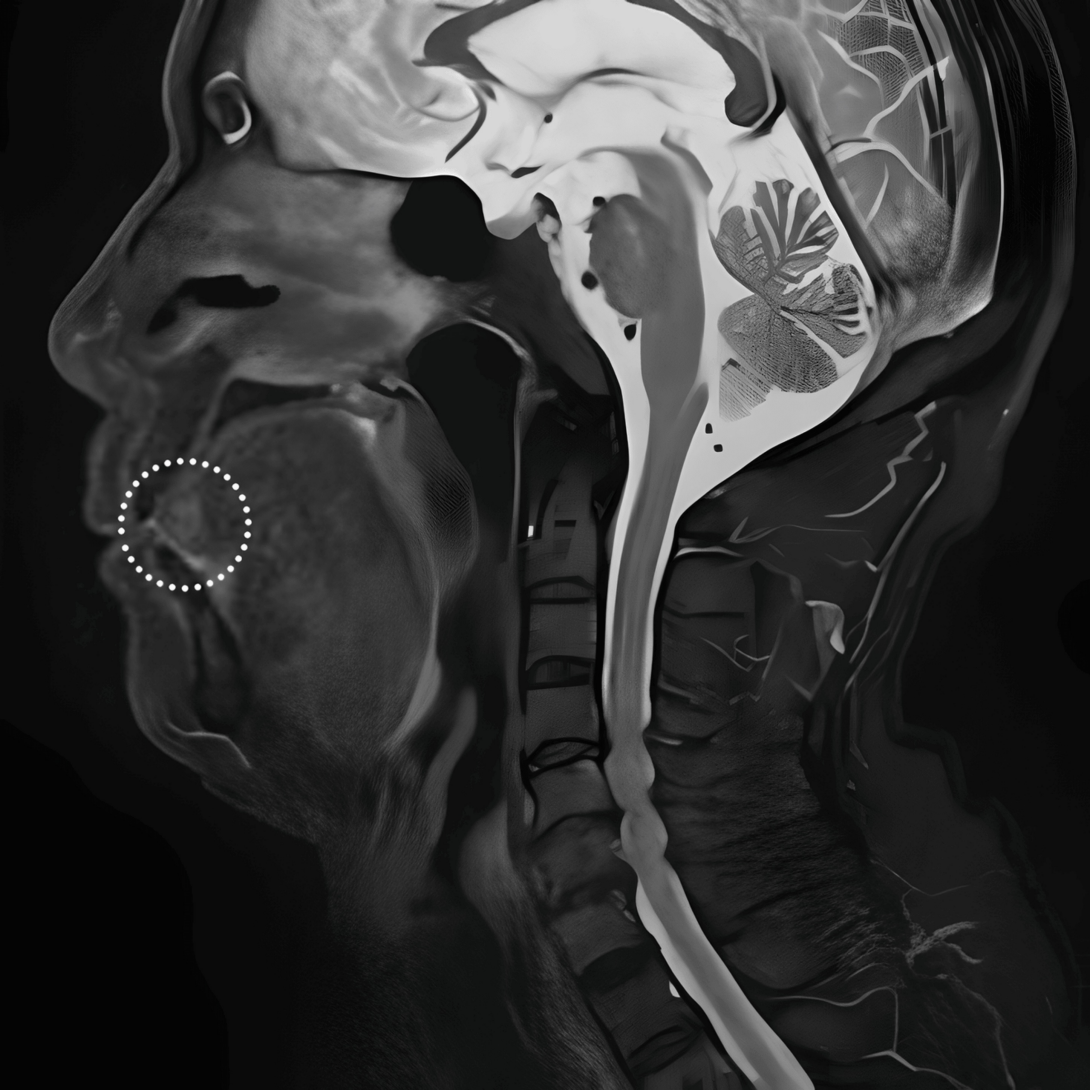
Magnetic resonance imaging The T2-weighted image shows a slightly high-signal area (dotted line) at the apex of the tongue.
